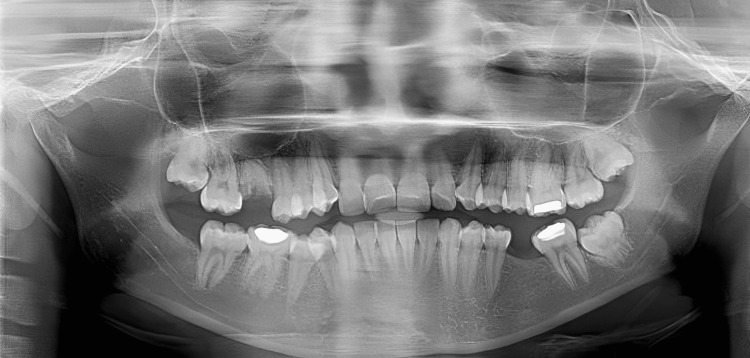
Preoperative OPG of the patient OPG: orthopantomography
